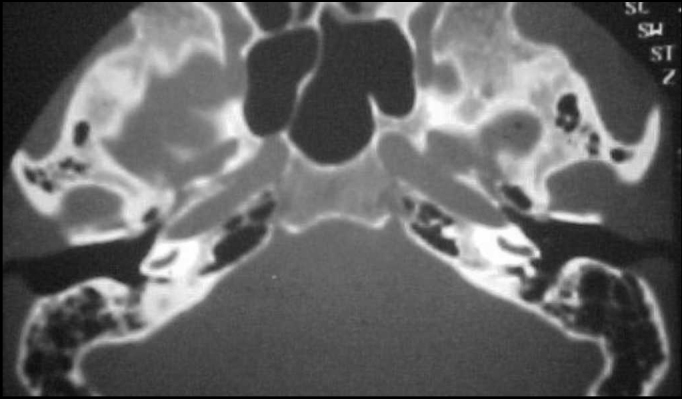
CT scan of temporal bone showing velamentum of the promontory and recess of the right facial nerve.
